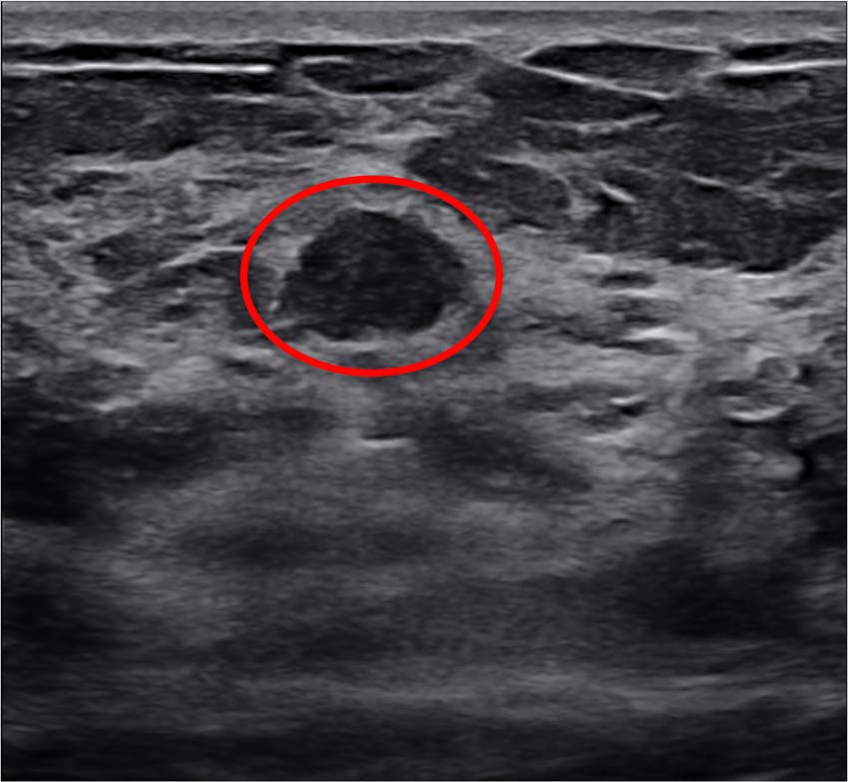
Ultrasound scan of the lesion (circled).
